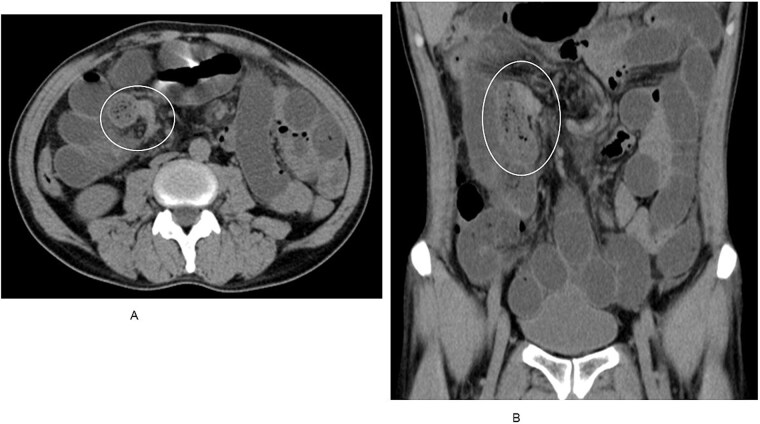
(A) Axial CT scan revealing a small bowel feces sign in the right upper quadrant. (B) Coronal CT view demonstrating collapsed distal bowel loops beyond the obstruction.
